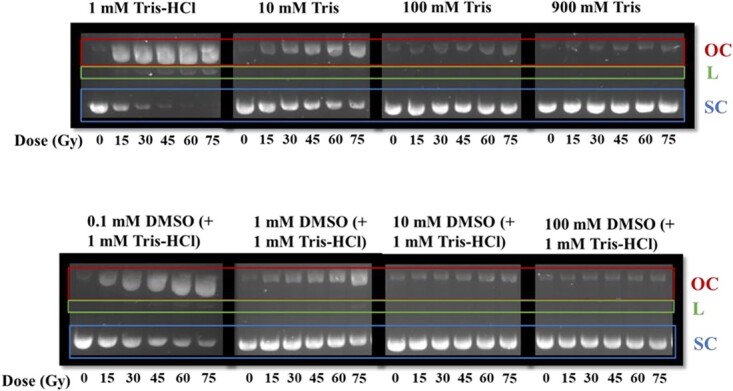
Image from agarose gels of X-ray irradiated samples where bands on the gel represent the pBR322 plasmid DNA. Plasmid DNA is separated out into three structures, SC (blue—bottom), OC (red—top) and L (green—middle), which are indicated by the coloured box surrounding the bands, and neighbouring labels. Image is original image as taken, with no post-processing. The dose (Gy) is indicated by *x*-axis labelling of each band below image, and the scavenger concentration is indicated by labelling above each box.
